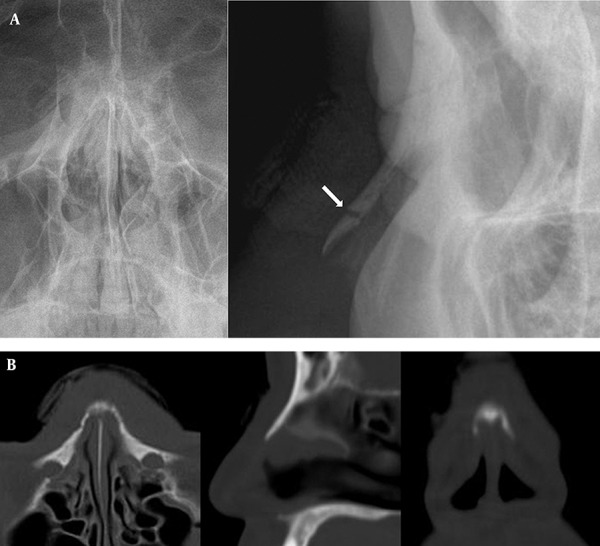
A 36-year-old man with painful nasal swelling and a simple non-depressed transverse nasal fracture. A, Coronal and lateral conventional radiography images show a discrete simple fracture in the mid-portion of the nasal bone. B, CT images show no discrete fracture on axial, sagittal, and coronal reformatted images (arrow indicates nasal bone fracture).
